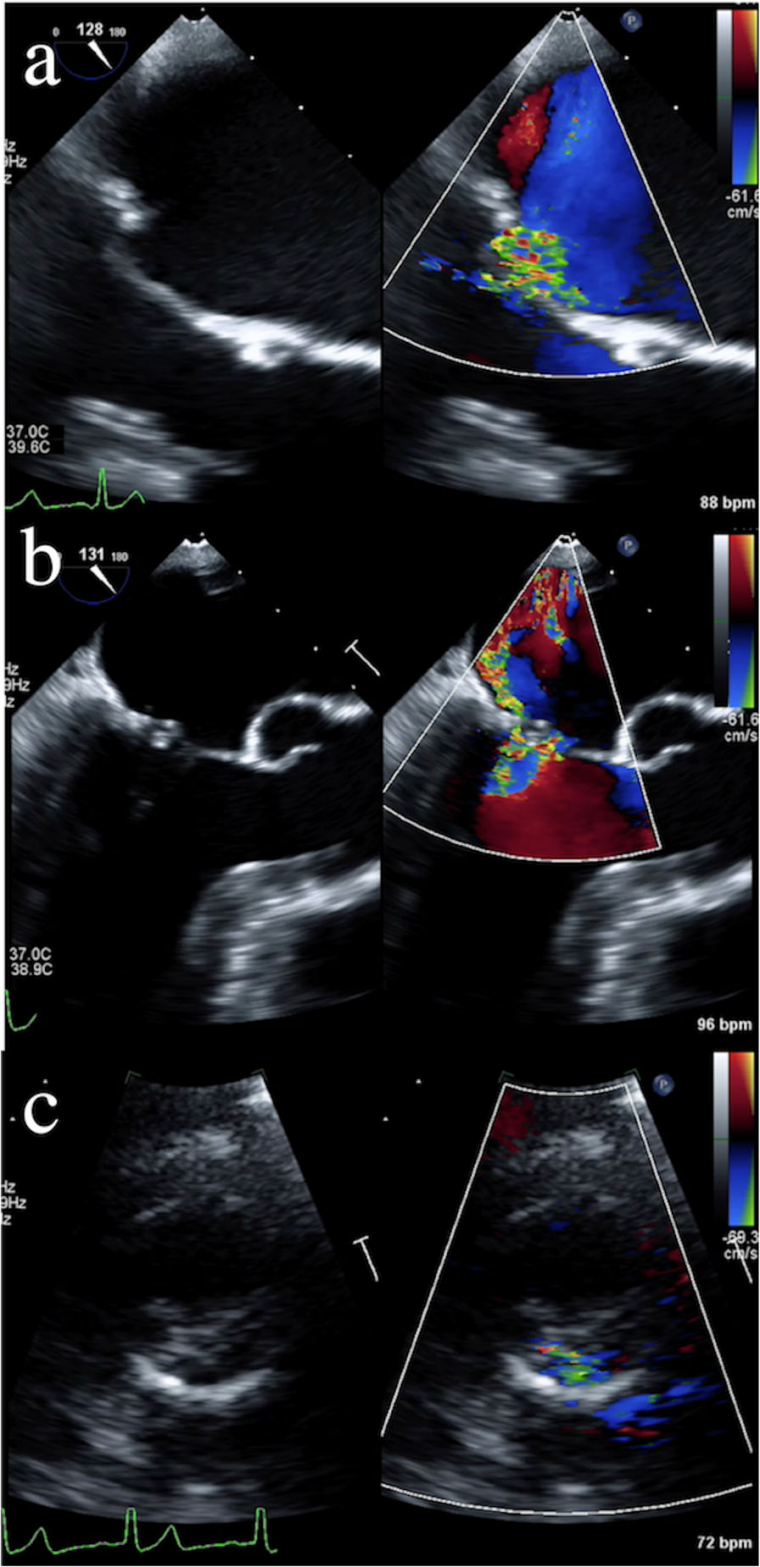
a Transesophageal echocardiography 8 days (early) after repair with neochordoplasty of A2 and quadrangular resection with sliding plasty of P2 showing anterior leaflet tethering and decreased motion of the posterior leaflet. b Transesophageal echocardiography 7 days (early) after repair with neochordoplasty of A2–3 showing A3 prolapse. c Transthoracic echocardiography 7 years (late) after repair with neochordoplasty of A2–3 and commissural plication of the posteromedial commissure showing a recurrent regurgitant jet at the posteromedial commissure
